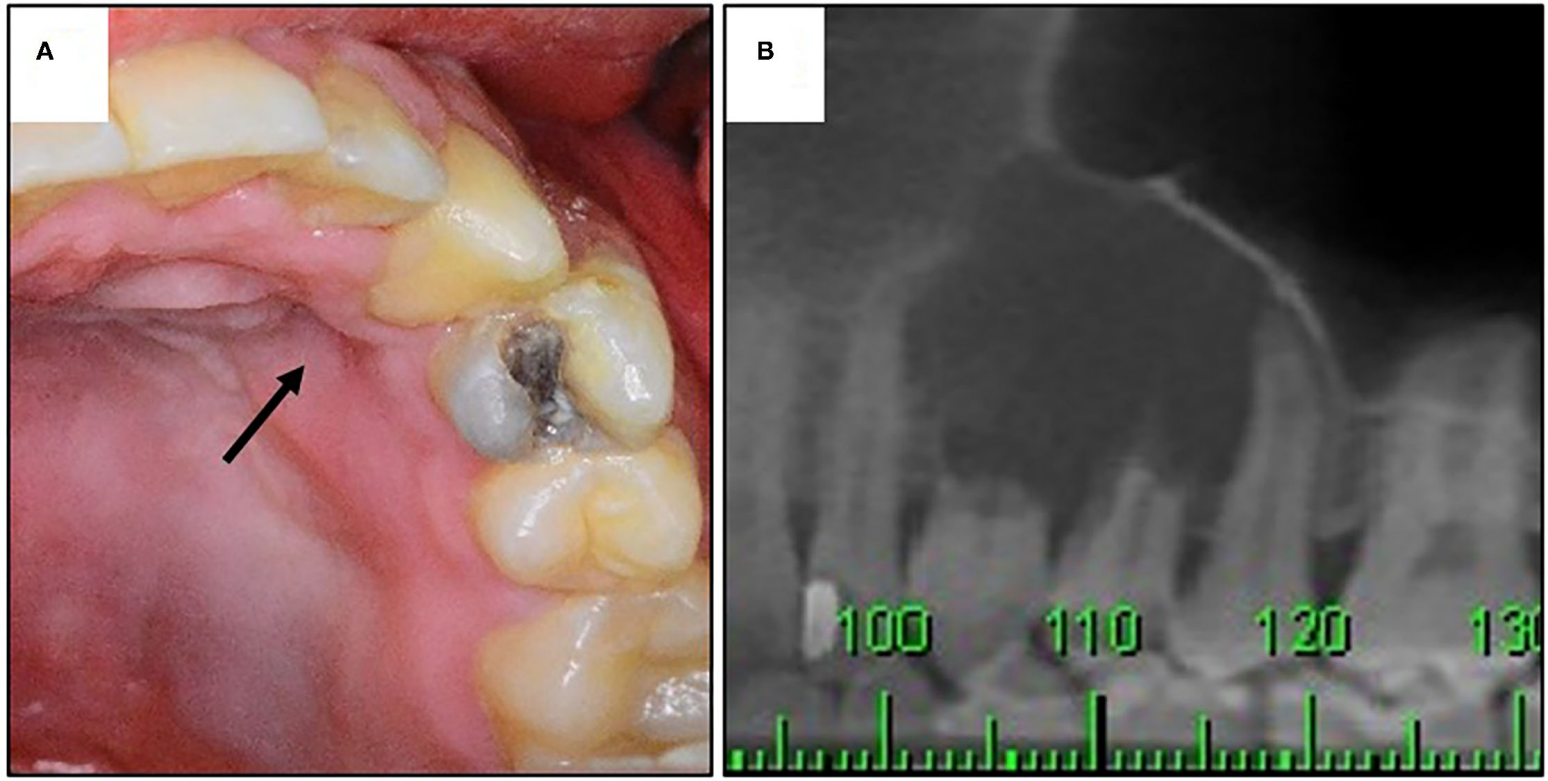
The clinical and radiographic findings of a patient with NCLC-CEOT. (A) Clinical picture showing palatal depression (arrow); (B) prominent root resorption in cone-beam CT (CBCT) image.
